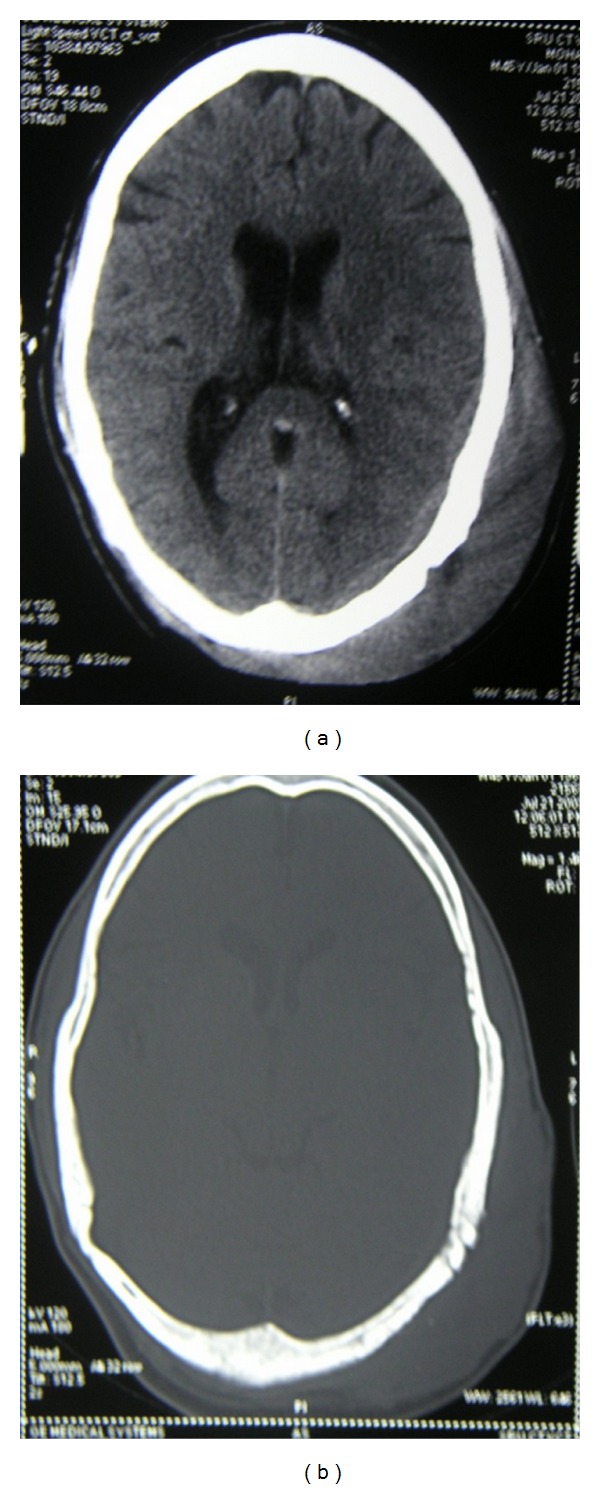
(a) CT scan axial section of the head showing scalp swelling in the left temporoparietal region after partial excision of the mass. (b) CT scan axial section showing scalp swelling after partial excision of the mass.
